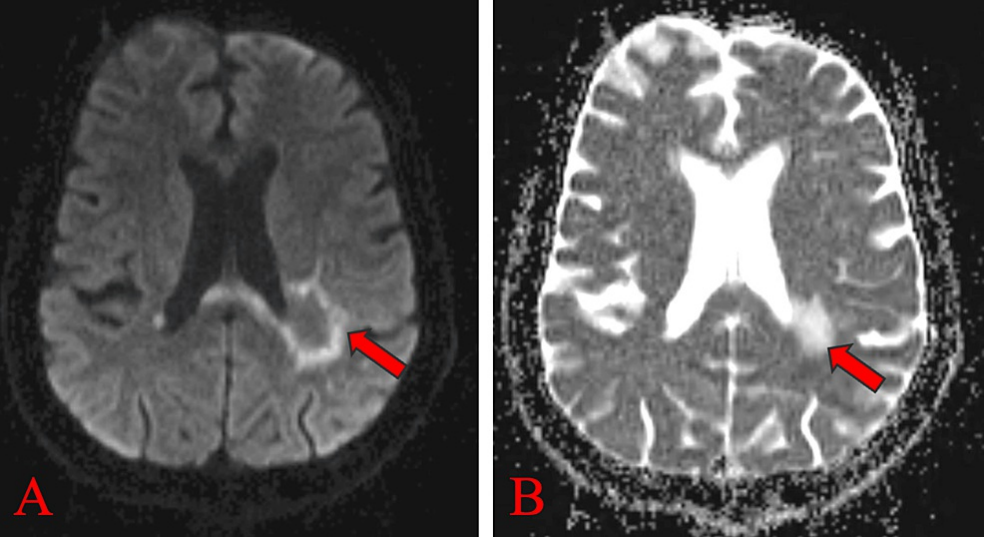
MRI brain (P+C) showing predominantly peripheral diffusion restriction on trace DWI image (A) with corresponding subtle hypointensity on ADC image (B) in the left peritrigonal region shown by red arrows. MRI brain (P+C): Magnetic resonance imaging brain plain and contrast; DWI: diffusion-weighted image; ADC: apparent diffusion coefficient
